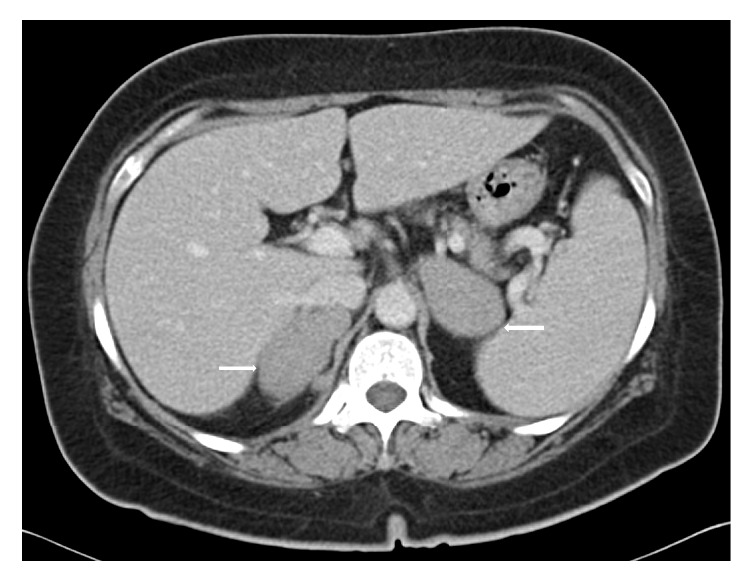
At the time of diagnosis. Imaging studies of patient, CT thorax, and abdomen, and pelvis show bilateral adrenal masses which are homogenous measuring 3.7 × 5.4 × 6.3 cm on the left (78.3 average HU), while the right adrenal gland measures 5.5 × 2.7 × 5.9 cm (72.7 average HU).
